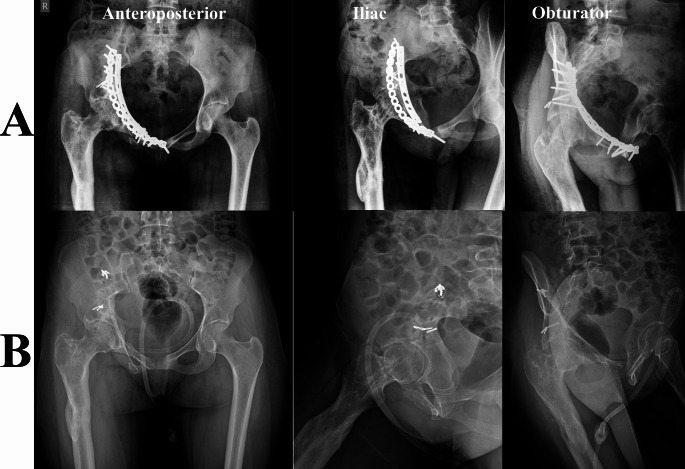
32-year-old female patient who had a T-type fracture treated by ORIF, hardware was performed through a modified Stoppa approach and a lateral window after 63 months of the index surgery. A, preoperative plain radiographs. B, postoperative. (Patient No. 7 in Supplementary File 2)
